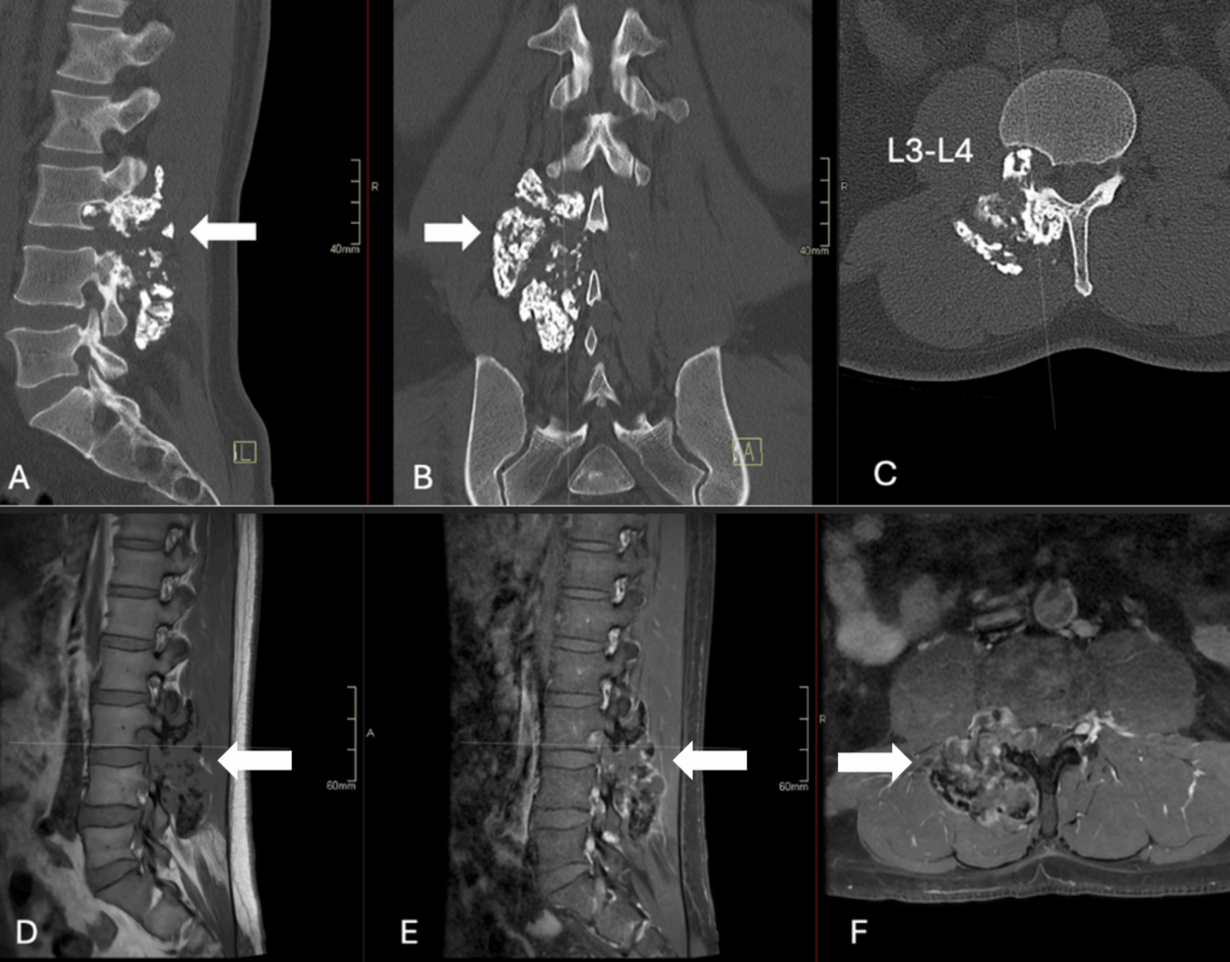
CT scans (sagittal (A), coronal (B), and axial (C) views); MRI (sagittal T1 (D), sagittal T1 Gd (E), and axial T1 Gd (F) views) The CT scan shows a right lumbar paraspinal mass between L3 and L5 with multiple clustered chondral calcifications and erosive involvement of the facet joints and L3 cortical rim. It occupies the L3-L4 foraminal canal (arrow in A and B). On MRI, it is seen as a polybulinous mass with heterogeneous signals on T1 and T2 with scant gadolinium enhancement. At L3, it shows slight compression of the dural sac to the left. Gd: gadolinium
